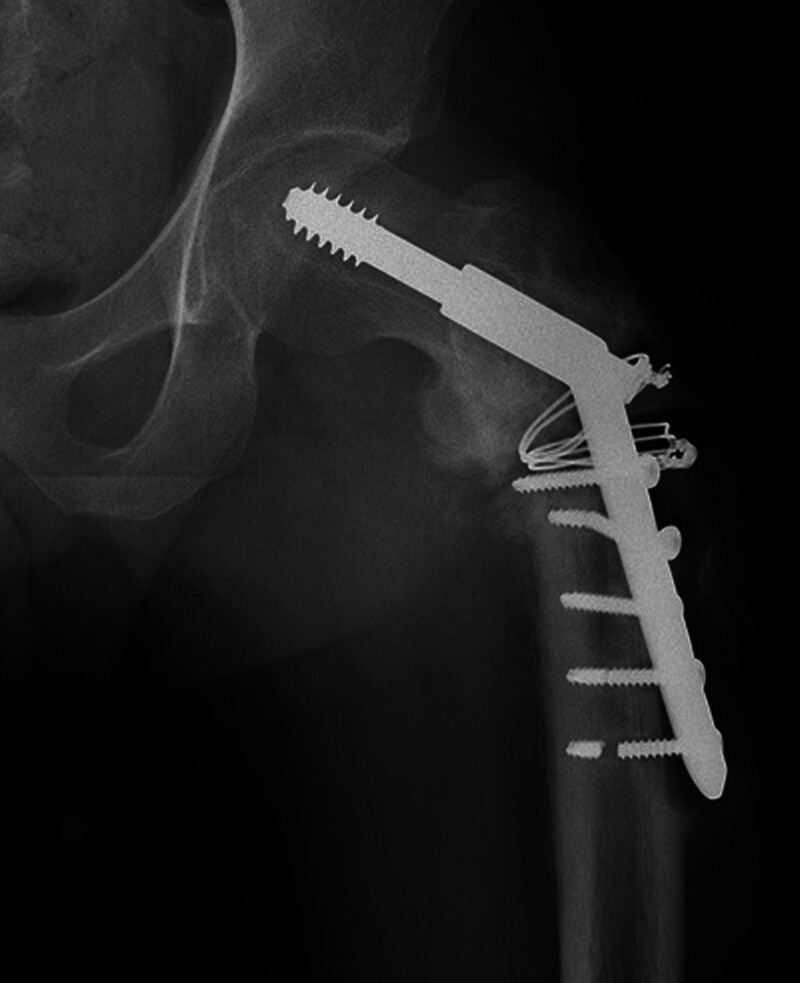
Radiograph displaying nonunion and implant failure of intertrochanteric fracture with broken screws.
